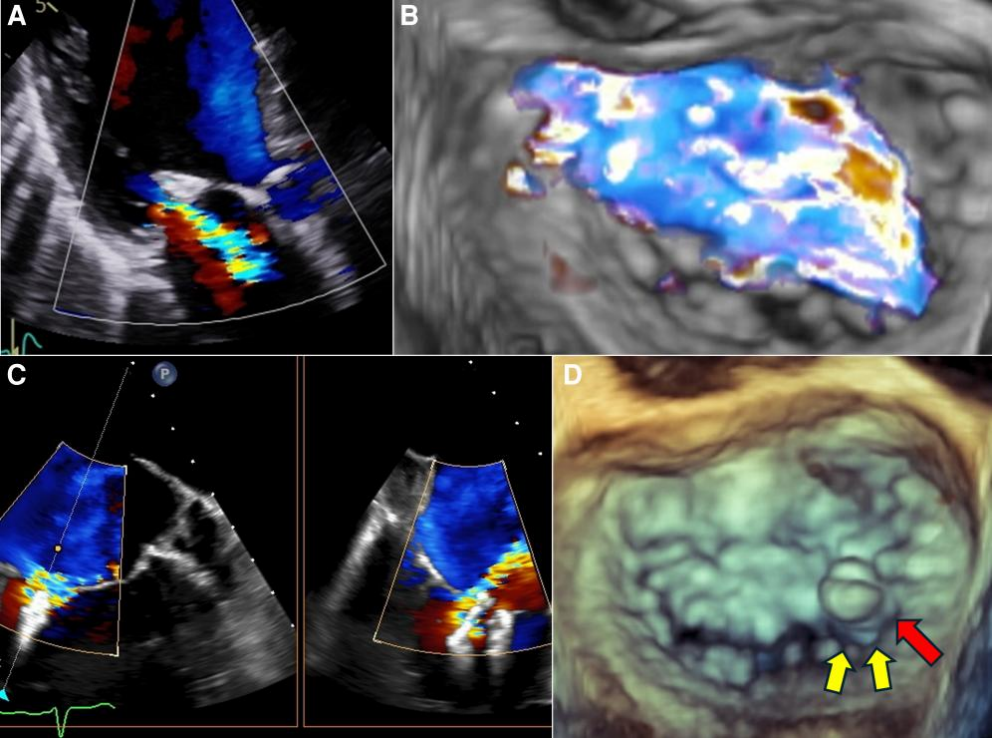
TTE and TEE images after the first procedure. Recurrent severe MR on TTE at 2 days post- transcatheter edge-to-edge repair (*A*). TEE confirming a leaflet tear and an MR jet posterior to the XTW clip (B–C). 3D-TEE showing a torn leaflet posterior to the XTW clip (yellow arrow) and a residual tissue bridge at the clip’s most medial part (red arrow) (*D*). MR, mitral regurgitation; TEE, transesophageal echocardiography; TTE, transthoracic echocardiography.
